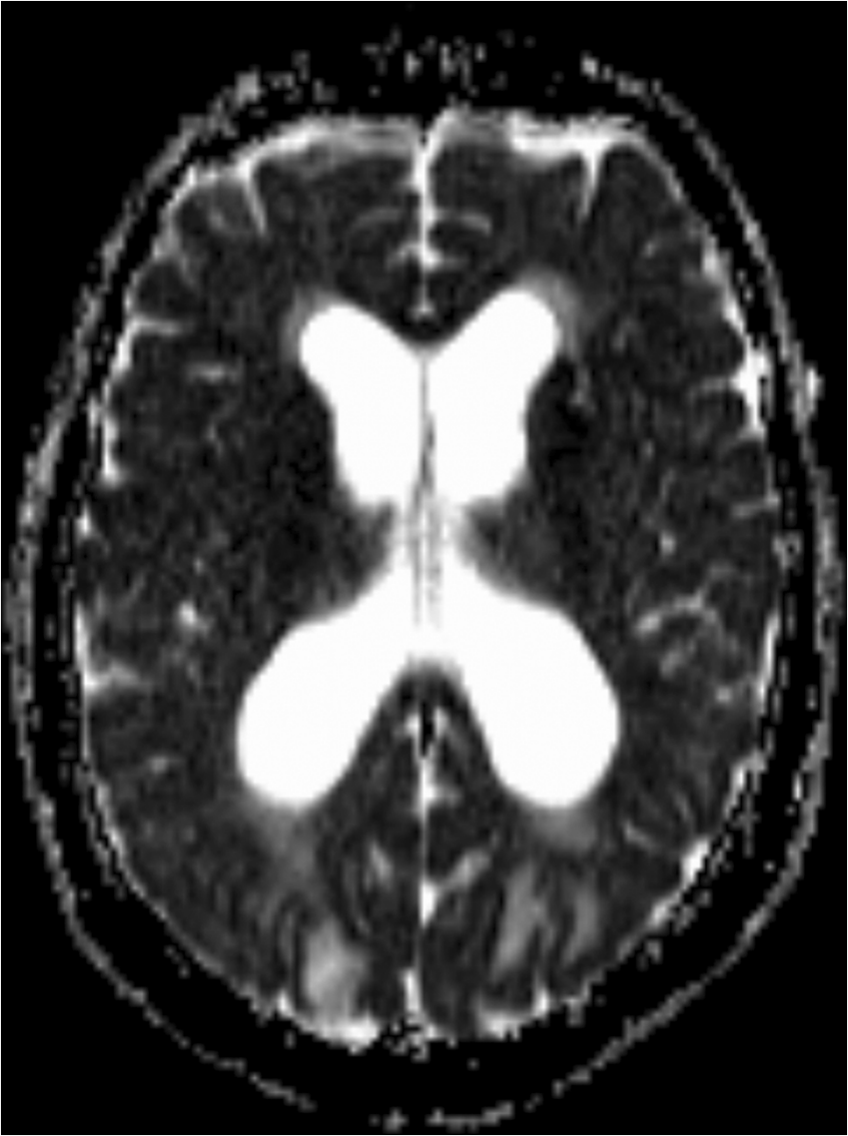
Brain axial apparent diffusion coefficient weighted magnetic resonance image shows hyperintensity in the bioccipital region.
